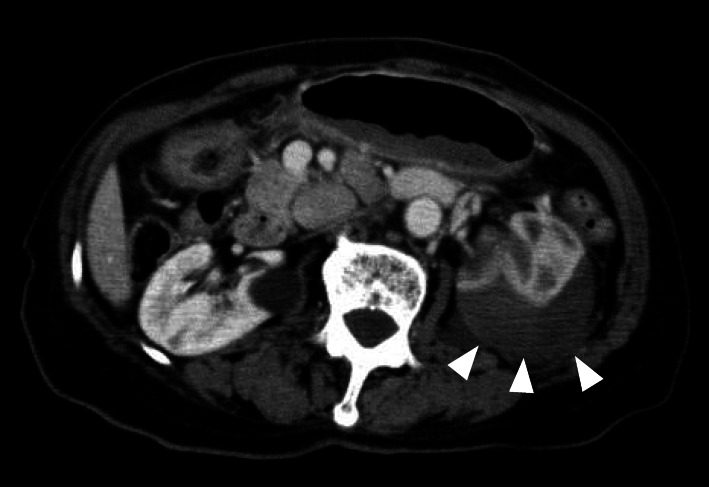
Plain CT image of the abdomen. Note the hematoma excluding the left kidney (arrow head)
